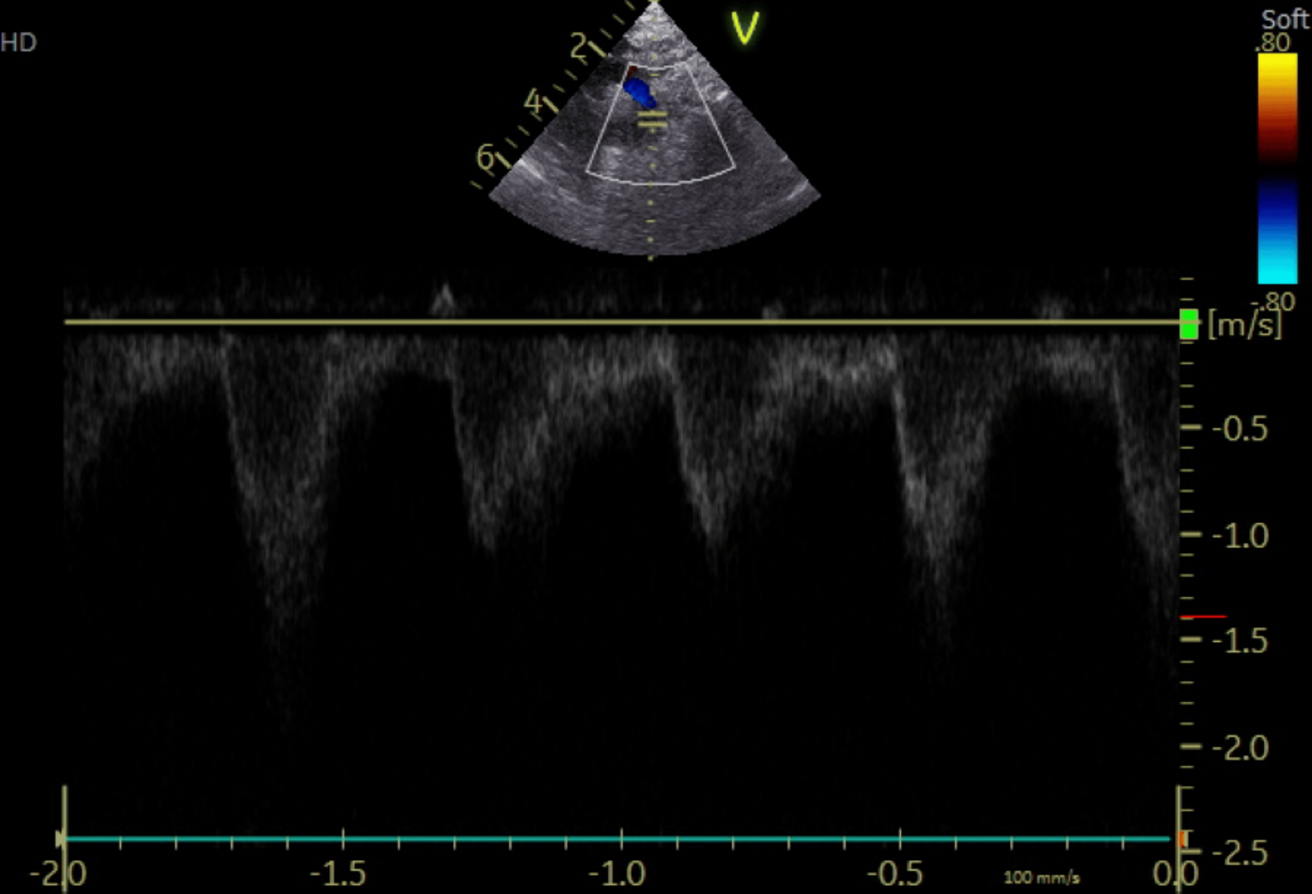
Echocardiography (2D/ Doppler) at approximately 36 hours of life revealed a patent ductus arteriosus with a left-to-right shunt and evidence of developing CoA flow (Case 2). CoA: coarctation of the aorta
